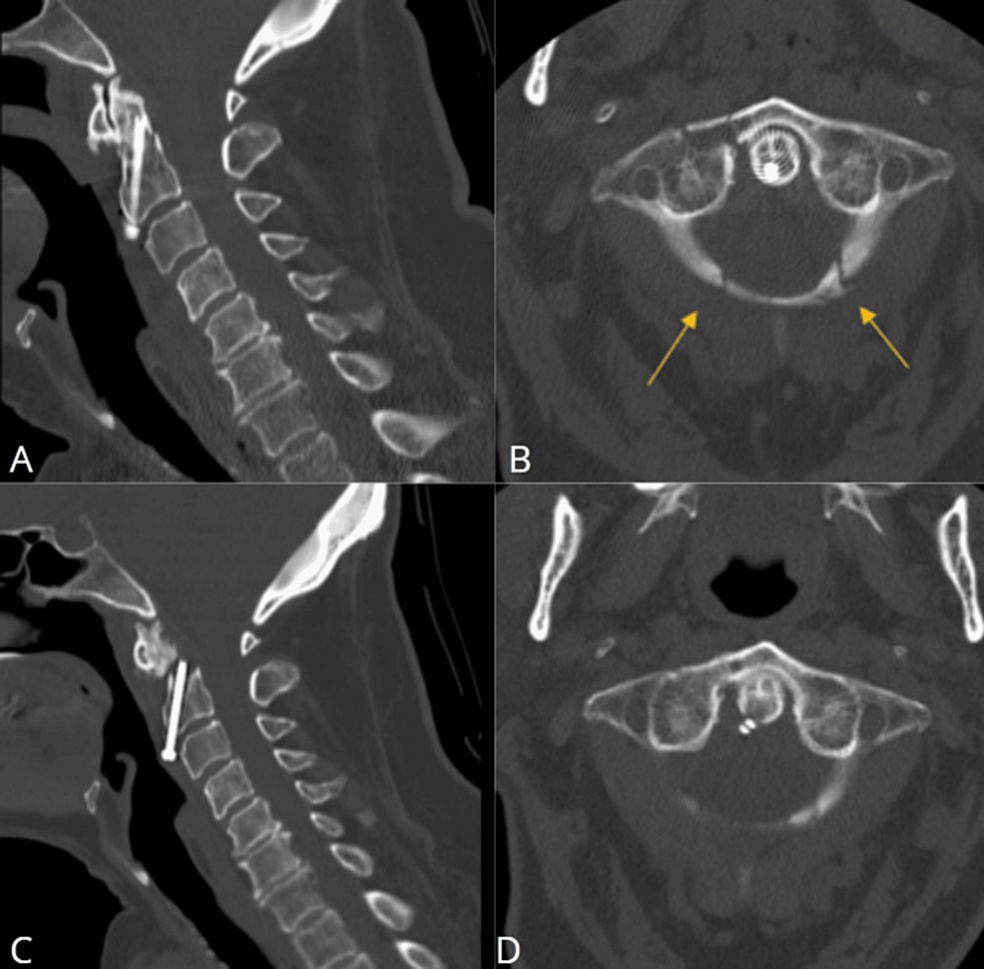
Cervical CT Good positioning of the anterior odontoid screw in sagittal view (A). Axial view (B) shows the tip of the screw and two fracture lines across the posterior arch of C1 (yellow arrows) not previously reported. Cervical sagittal CT demonstrated a single intact screw traversing the C2 vertebral body; however, the screw no longer contacted the dens (C). Another view in the axial image (D).
